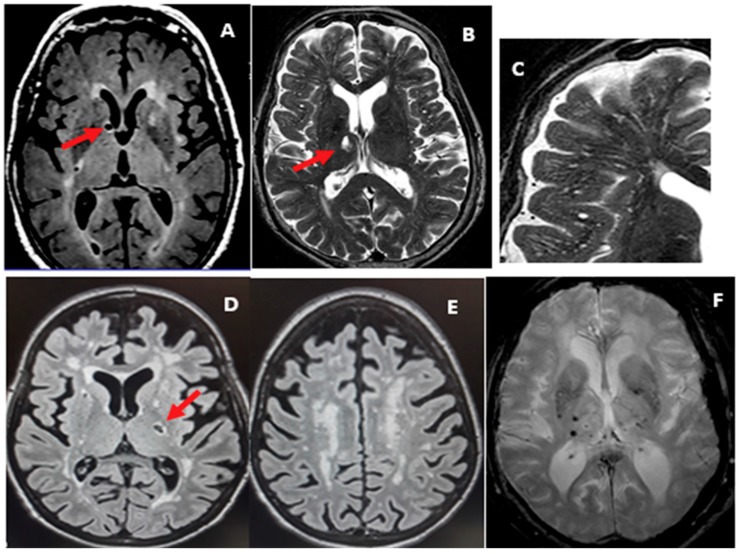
MRI, patients with cerebral small vessel disease and cognitive impairment/dementia (A–F). (A,B,D) lacunes (red arrow): are seen in FLAIR (Fluid Attenuated Inversion Recovery) as a cavity hypointense, with hyperintense halo, between 3 mm and 15 mm diameters (A,D) and on T2 as hyperintense signal similar to cerebrospinal fluid (B). Enlarged Virchow–Robin perivascular spaces (C) follow the course of a vessel through grey and white matter. White matter hyperintensities on T2-weighted images (B,C) and FLAIR (D,E), but without cavitation. The lesions are confluent around the ventricles and subcortical white matter (Fazekas III) and the signal differs from cerebrospinal fluid. (E) T2*-weighted MRI shows two microbleeds, areas of void signals in the right basal ganglia.
